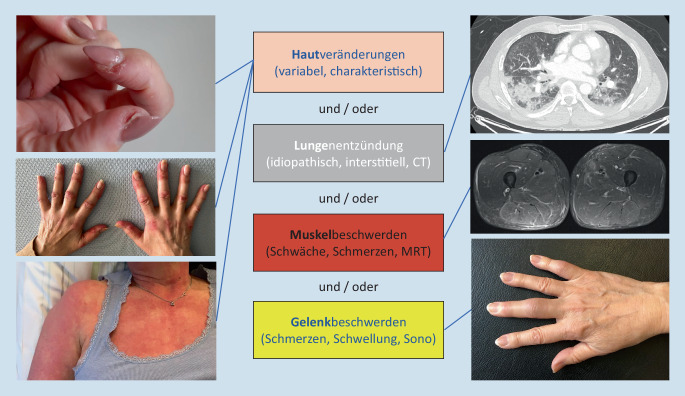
Selbstverständlich ist, dass primär auch alle anderen und oft häufigeren Differenzialdiagnosen für die vorliegende Symptomatik adressiert werden. Auch sei an dieser Stelle darauf hingewiesen, dass die Autoantikörperbestimmung nur ein „Diagnostikbaustein“ ist und in der Abklärung einer IIM je nach Manifestation eine Biopsie von Haut, Muskulatur oder Lunge und eine Bildgebung mittels MRT der Muskulatur oder CT der Lunge diagnostisch wertvolle Informationen liefern können.
